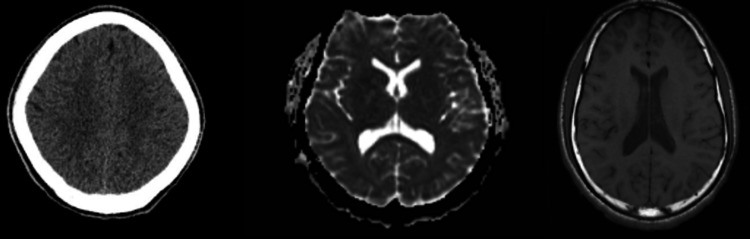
(Left) Non-contrast CT brain showing no evidence of acute intracranial hemorrhage, mass effect, midline shift, or hydrocephalus. (Center) MRI brain without contrast demonstrating normal parenchymal signal intensity on T1- and T2-weighted sequences, with no signs of acute infarct, mass lesion, or demyelinating process. (Right) MRI brain with contrast showing no abnormal enhancement.
